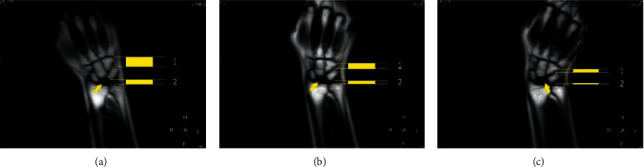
Multiangle comparative analysis of the ulnar deviation motions of the wrist of the patient using MRI: (a) radial deviation motion of approximately 10°, (b) ulnar deviation motion of approximately 5°, and (c) ulnar deviation motion of approximately 20° (arrow points to the necrotic lunate, showing the black area in the image. Distance 1 indicates the change in relative position between the uncinate bone and triangular bone. Distance 2 is the change in the relative position between the triangular bone and ulna).
